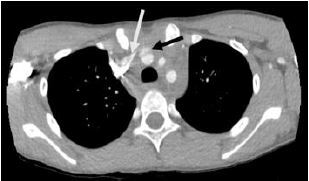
Pseudoaneurysm of the innominate artery (black arrow) seen in the transverse axis on computed tomography angiogram imaging with contrast. Mediastinal hematoma (white arrow) is seen layering anterior to the hyperdense subclavian vein as contrast extravasates out of the ruptured pseudoaneurysm.
